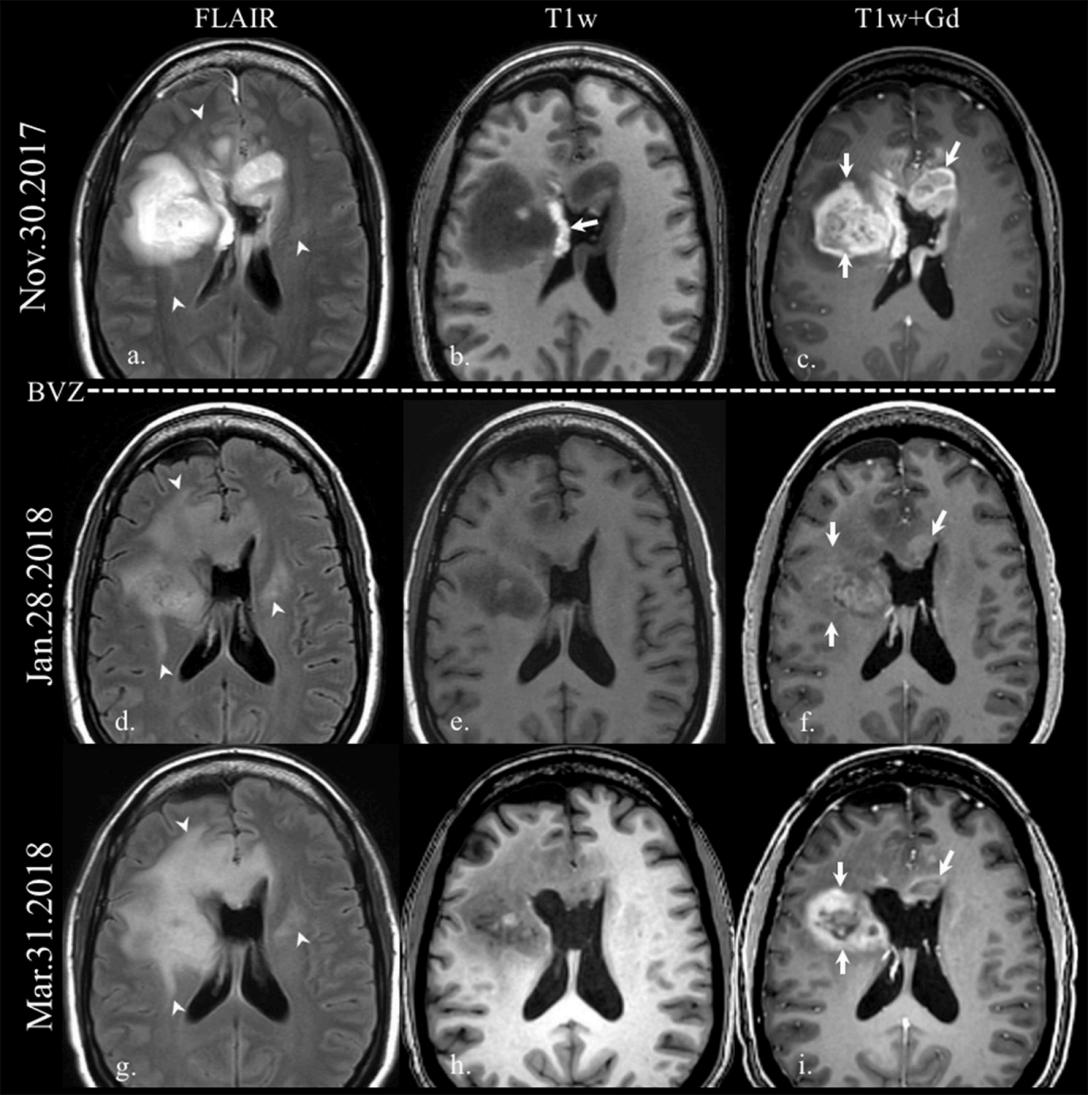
Enhancement in pseudoresponse. Brain MRI of a old patient with a GBM (IDH wild-type, MGMT status unknown) on the first recurrence after standard-of-care treatment. Axial FLAIR (a,d,g), and axial T1w before (b,e,h) and after gadolinium administration (c,f,i) images are displayed. On the top row, the immediate postoperative scan after the second resection demonstrates residual enhancing tumor on the right frontal lobe and on the left aspect of the genu of the corpus callosum (arrows on c). The arrow on b points some post-surgical blood products on the lateral wall of the right lateral ventricle. Note how the FLAIR hyperintensity extension increases on follow-up scans (arrowheads on a,d,g) after the onset of treatment with bevacizumab (BEV), while the enhancing area decreases dramatically (arrows on f), with reappearance despite antiangiogenic treatment (arrows on i).
